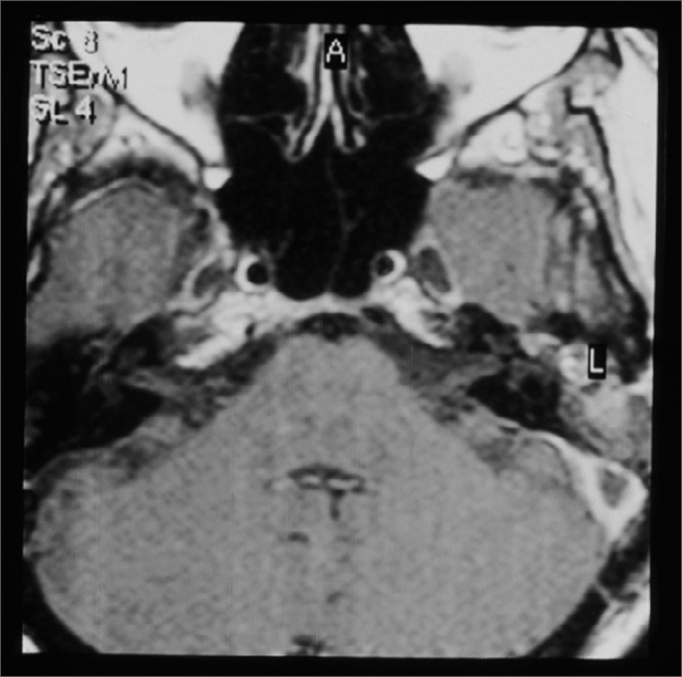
Temporal bone contrasted MRI, showing contrast highlight around the sigmoid sinus and no highlight inside, suggesting that there is a thrombus inside.
